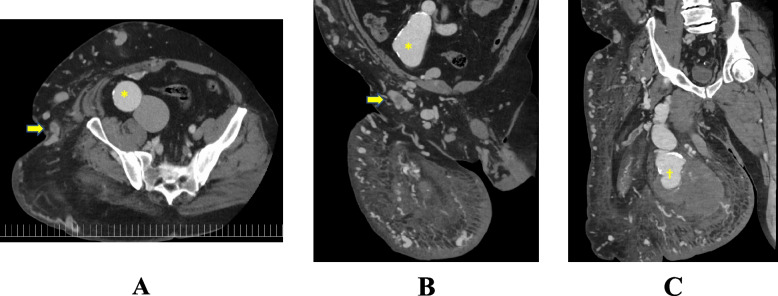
Preoperative CT image. A Transverse plane: an external iliac artery aneurysm (*), numerous collateral vessels, dilatation of vessels, and venous thrombosis (arrow) were observed. B Coronal plane: an external iliac artery aneurysm (*), numerous collateral vessels, dilatation of vessels, and venous thrombosis (arrow) were observed. C Coronal plane: a superficial femoral artery aneurysm (†) and numerous collateral vessels were observed
